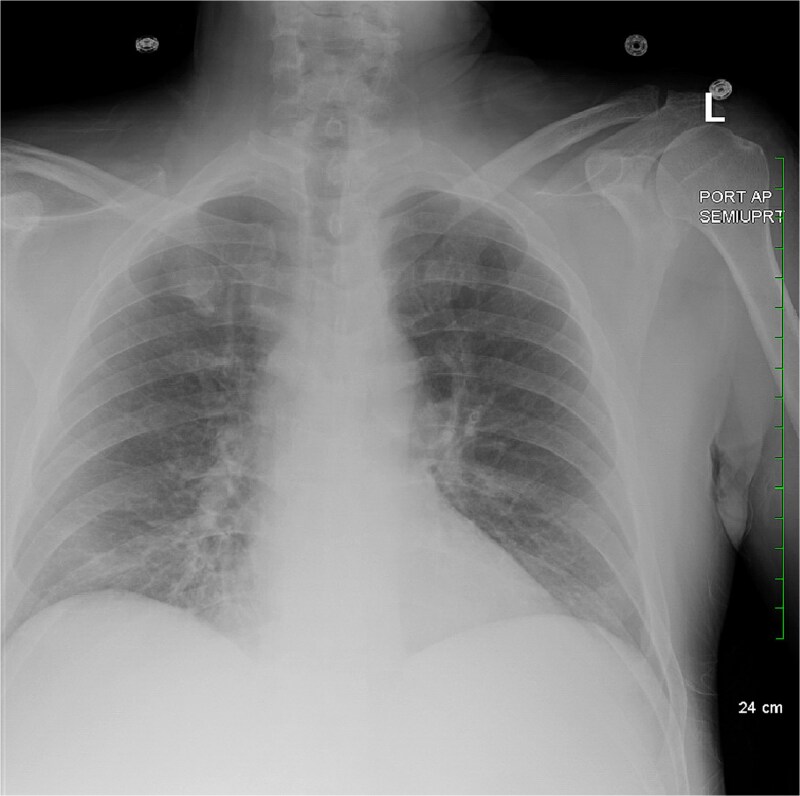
Pre-operative chest X-ray (anterior–posterior).
